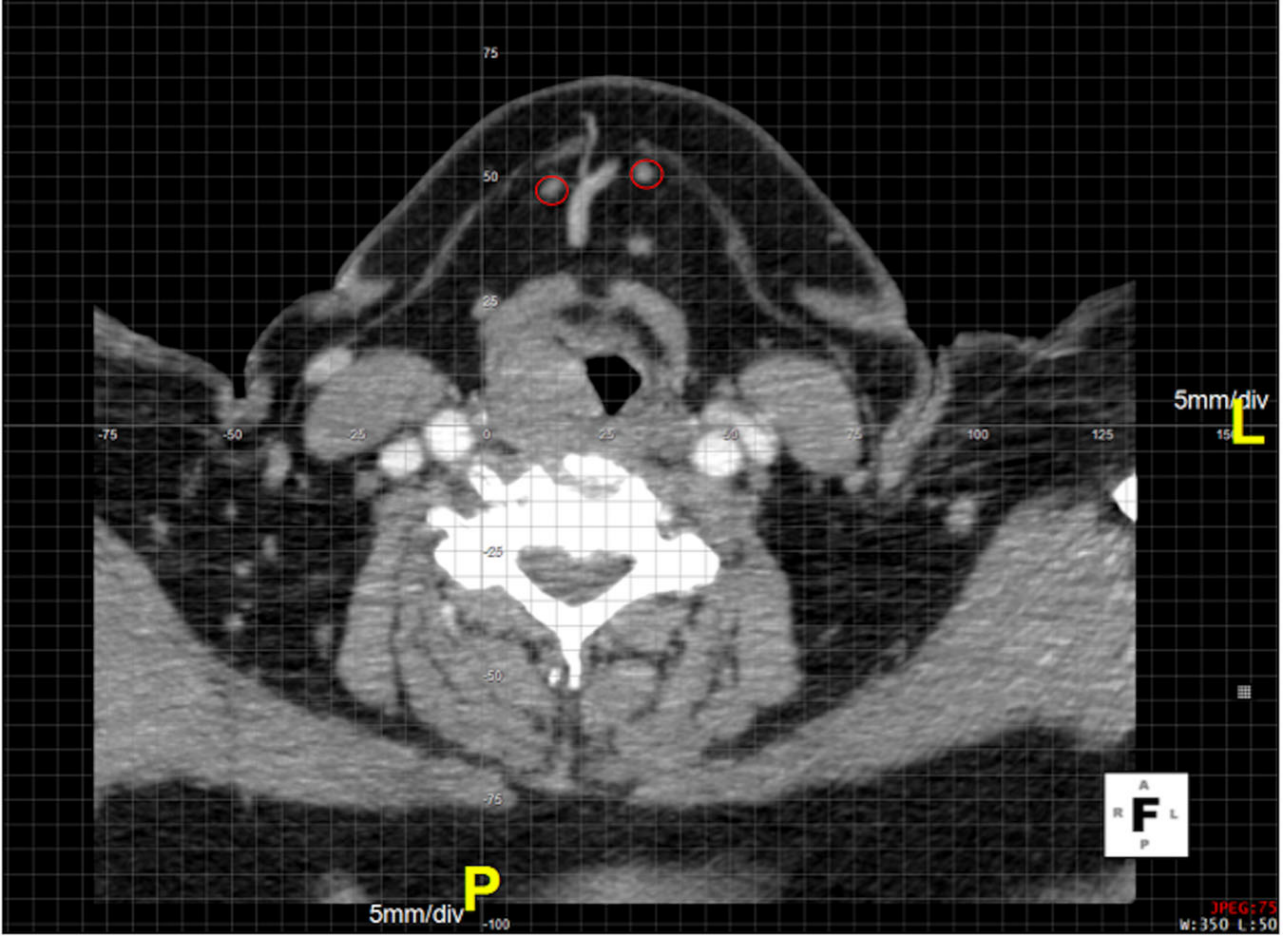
Axial slice of a head/neck CTA scan. The origin of the right submental artery is centered in the coordinate system. Two lymph nodes encircled in red. CTA, computed tomography angiography
